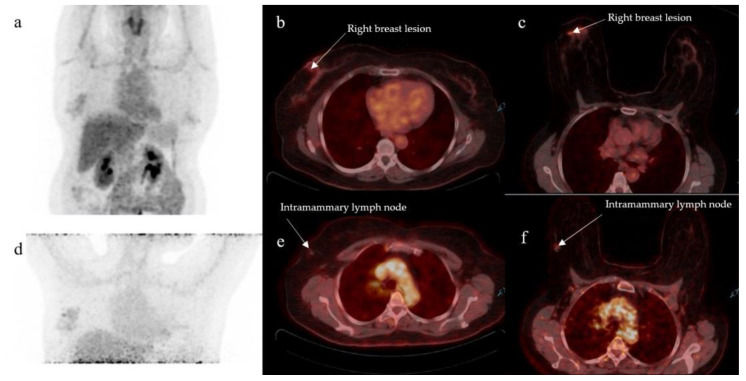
MIP images (a,d) showing uptake in the right breast. Fused axial PET/CT cuts showing faintly FDG-avid on supine right breast lesion (b), which is better visualized on prone (c). An intramammary lymph node appears non-radiotracer avid on supine (e) while it appears avid on prone (f).
